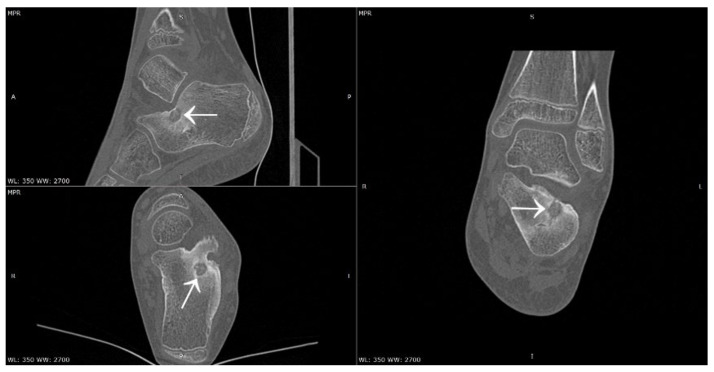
CT scans showing a focus of OO (arrows) in the left calcaneus.
